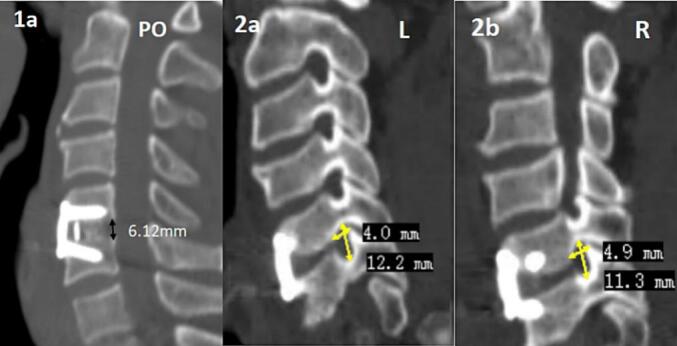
Post-revision Surgery CT:(1a) Post-revision posterior disc space height(2a) Post-revision left foramen height and width(2b) Post-revision right foramen height and width.
